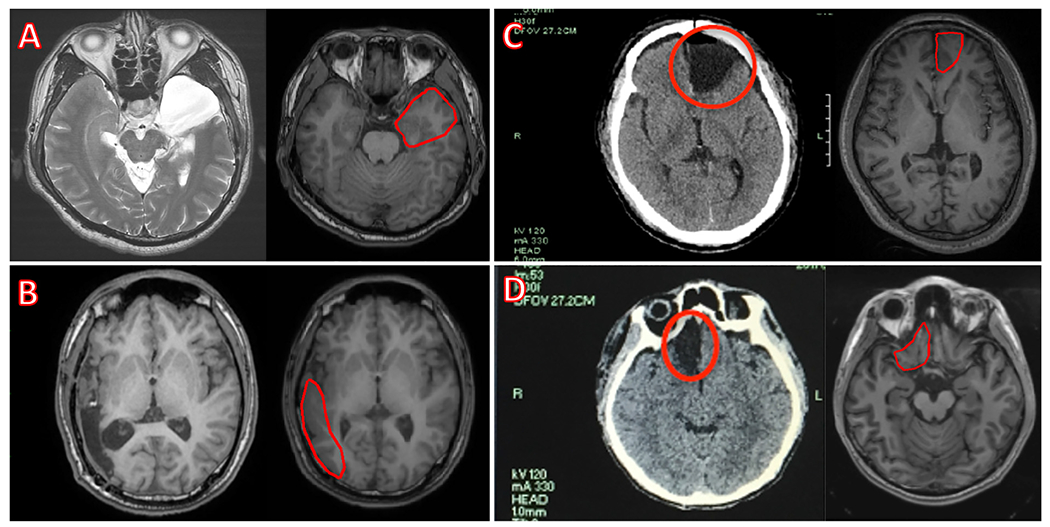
Examples of the volume of interest (VOI) marking process. VOIs marked on the pre-surgical magnetic resonance image (MRI) (the right figure of each pair of images) based on the post-surgical MRIs for (A) subject S5 and (B) subject S9; or on the post-surgical CT images for (C) subject S7 and (D) subject S6 are shown.
